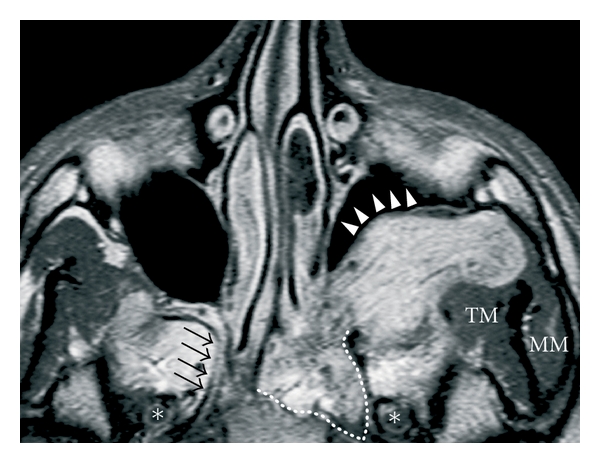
Axial contrast enhanced MRI: extensive JA with a typical pattern of spread into the cancellous bone of the basisphenoid along the vidian canal (white dotted line); on the contralateral side, black arrows indicate the right vidian nerve. Moreover, the lesion spreads deeply into the pterygomaxillary fossa toward the masticatory muscles, with anterior displacement of the posterior maxillary wall (white arrowheads). Asterisks indicate the foramen ovale bilaterally. TM: temporalis muscle; MM: masseter muscle.
